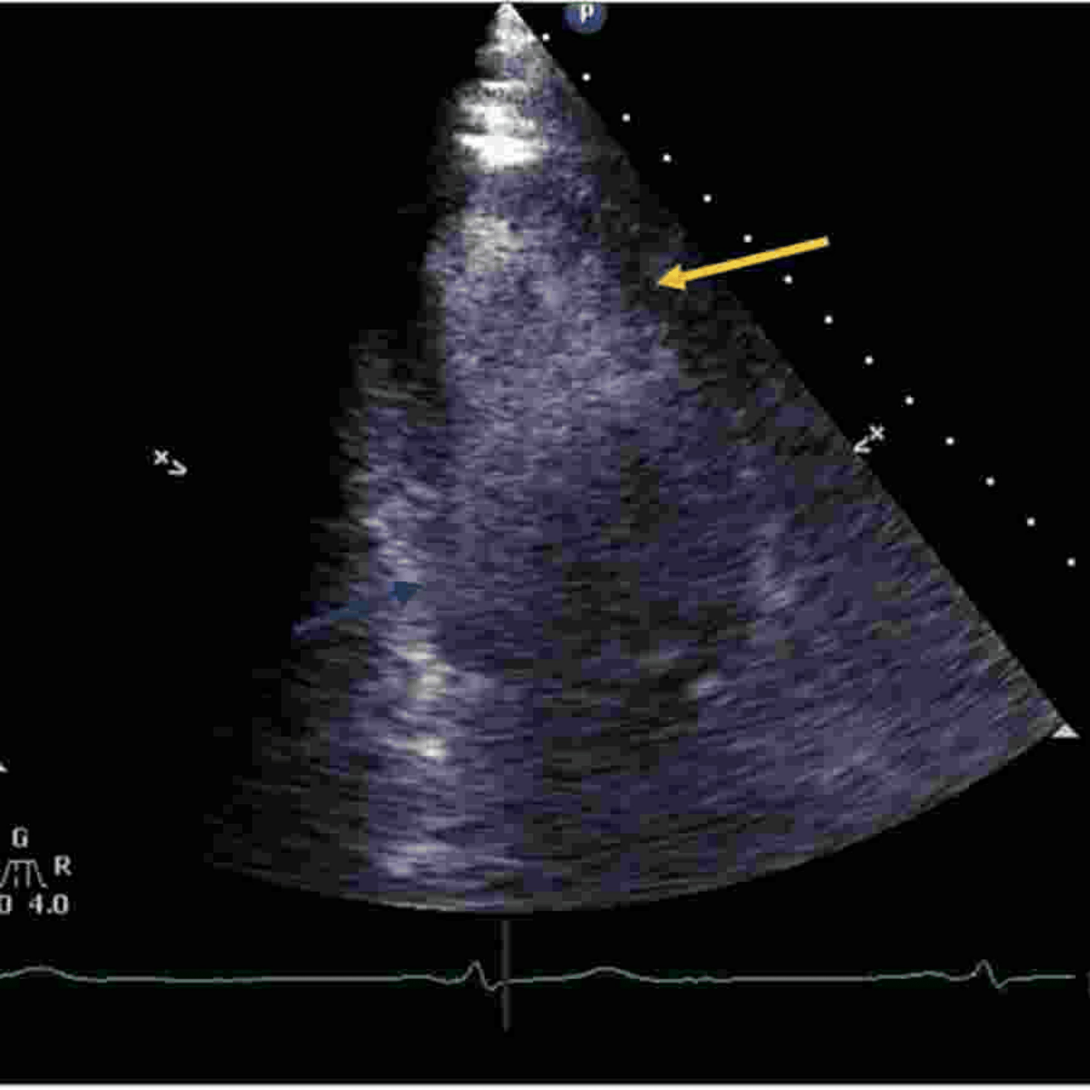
In this contrast TTE apical view of the left ventricle during systole, the yellow arrow indicates apical contraction of LV during systole, but at the same time blue arrow shows hypokinesis of the base of LV. There is some baseline chronic apical hypokinesis secondary to previous myocardial ischemia, but hypokinesis of the base of LV is prominent. LV: Left Ventricle, TTE: Transthoracic Echocardiogram
